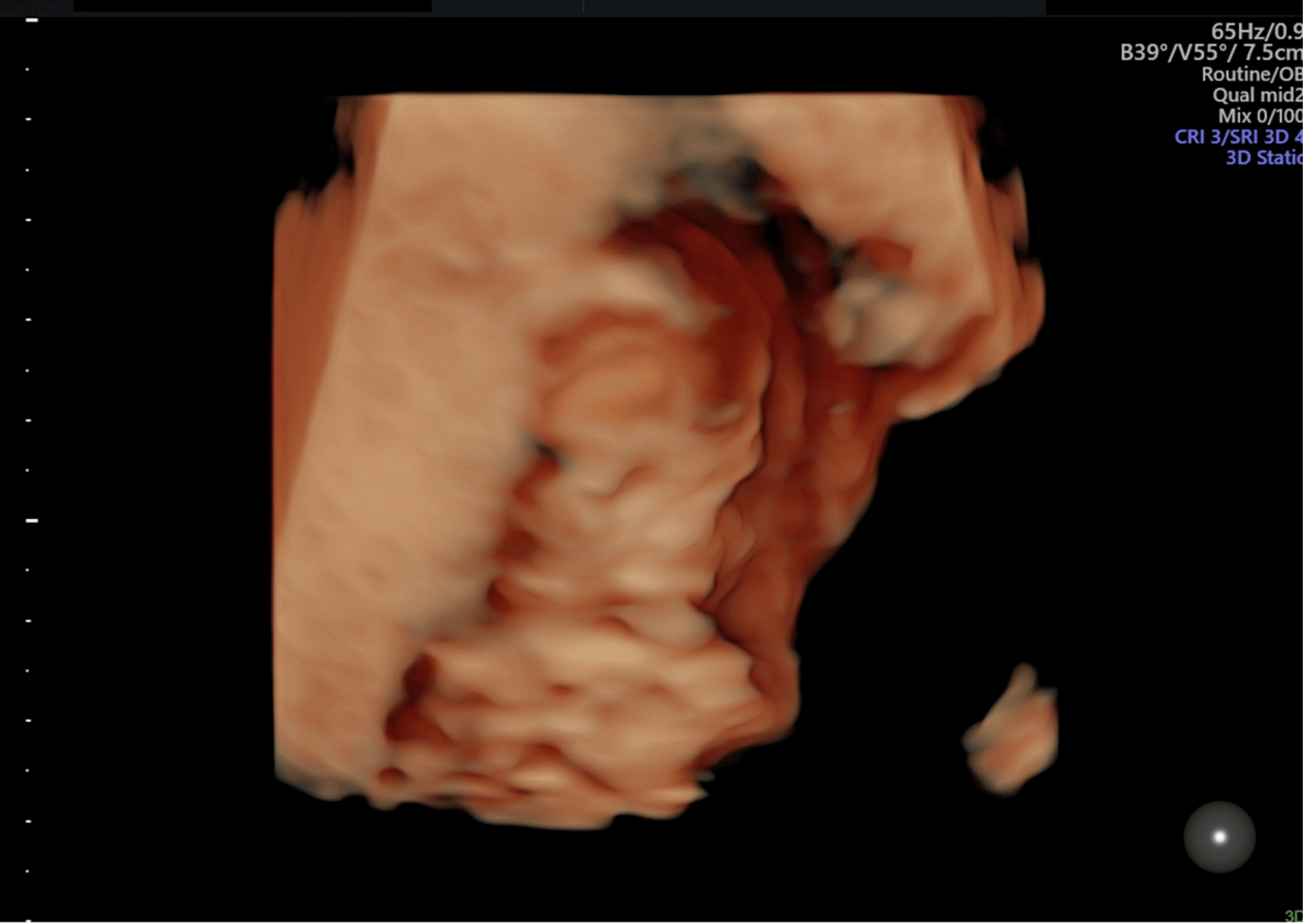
Antenatal ultrasound around the 17th week; three-dimensional rendered image of the fetal face shows normal features
